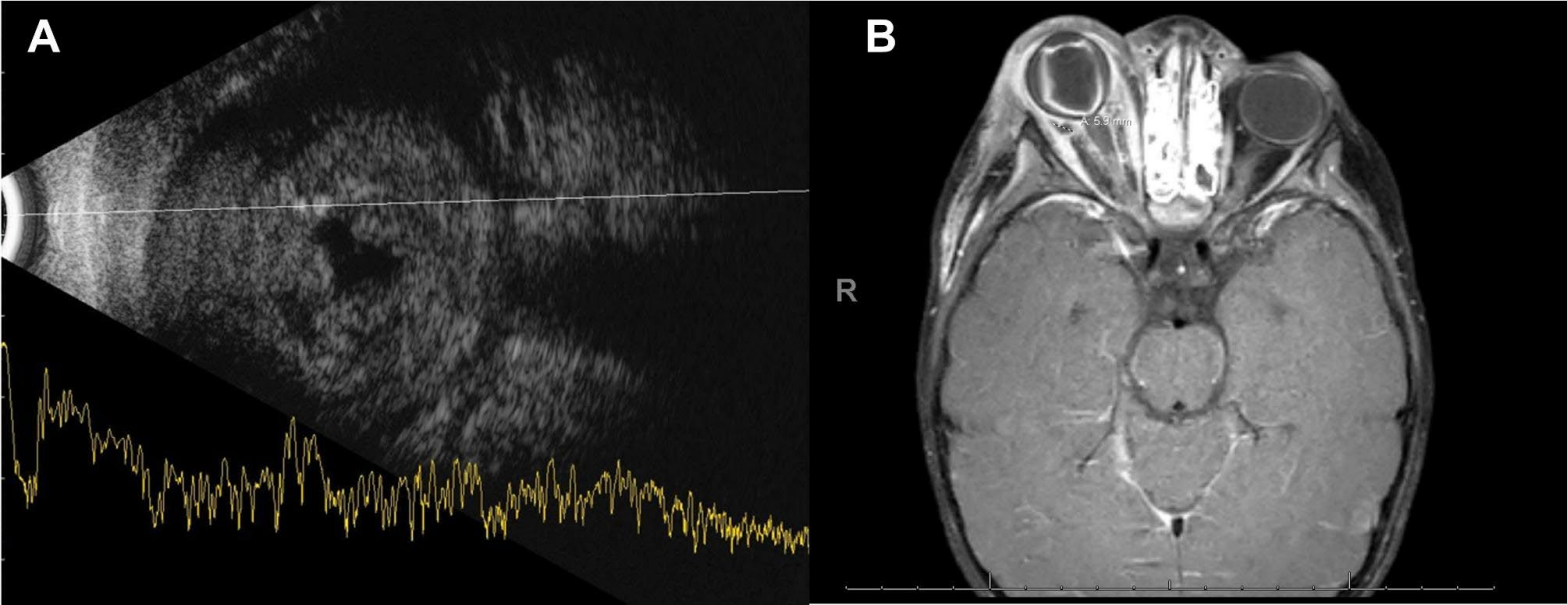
(A) Ocular ultrasound showing total retinal detachment with exudates. (B) MRI showing right globe proptosis with tenting of the posterior globe and stretched appearance of the optic nerve. There is abnormal vitreous signal with retinal detachment. Severe paranasal sinus mucosal disease was also noted
